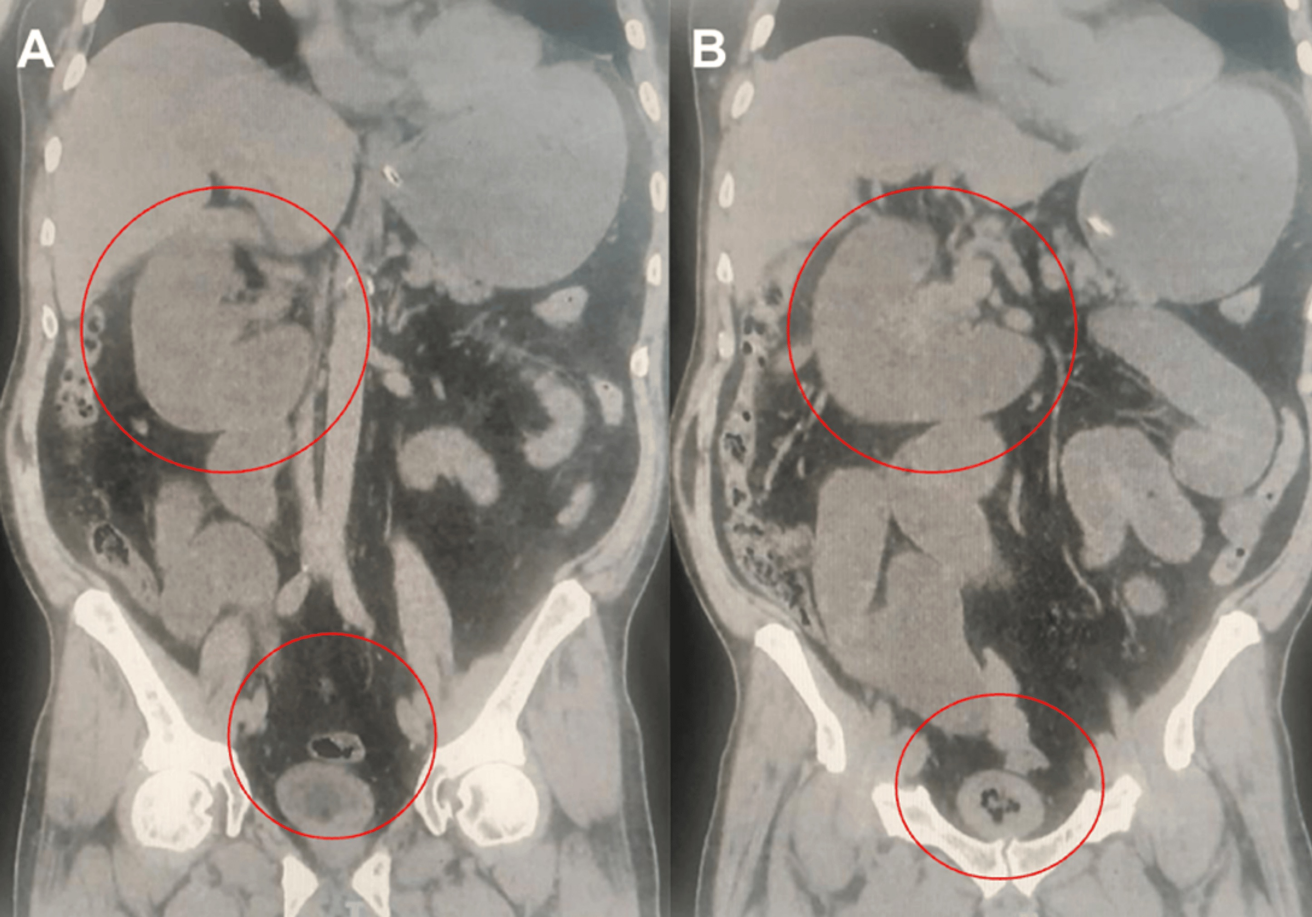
Coronal projection of abdominal CT scans (A) (B) Evidence of small bowel obstruction, inflammatory changes in the right kidney, and emphysematous cystitis
